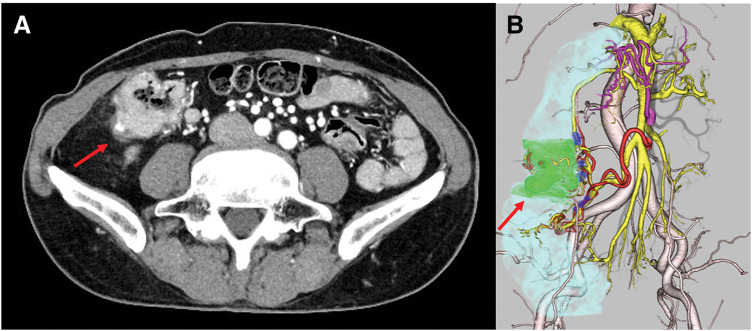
Findings of contrast-enhanced abdominal CT (A) and 3D reconstructed CT (B). Contrast-enhanced abdominal CT and 3D reconstructed CT revealed an enhanced mass in the ascending colon with a fat stranding sign near the hepatic fold (red arrow).
